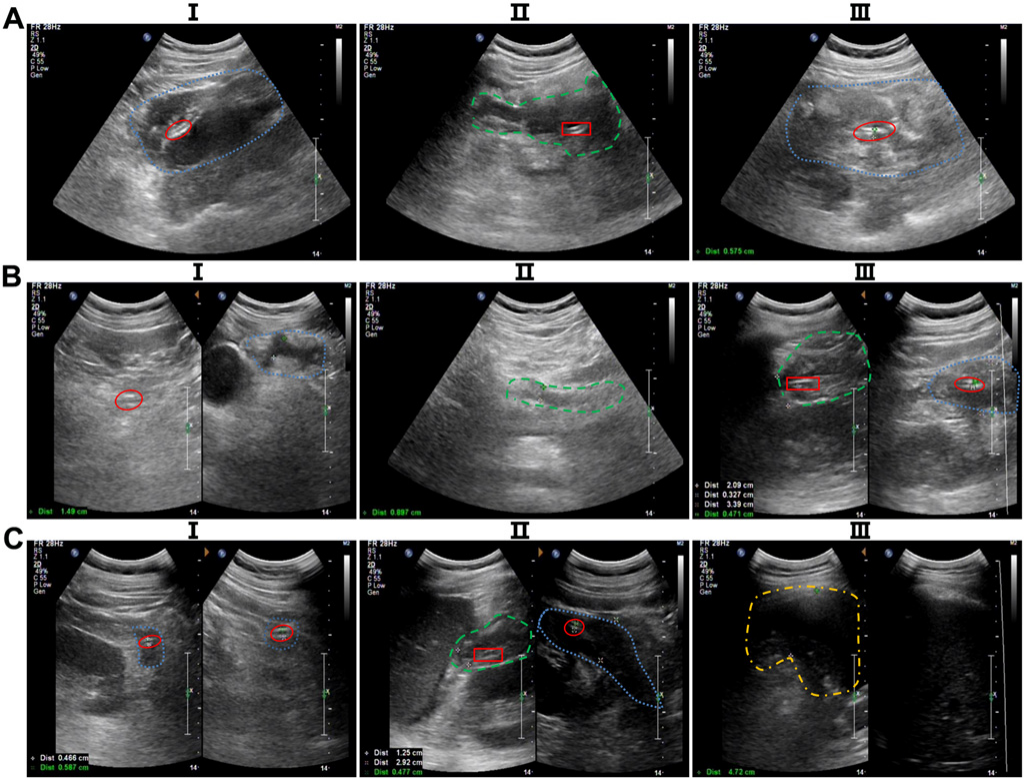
Ultrasonic images of fluid collections and drainage catheters at different time intervals after the interventions. (A) One day after drainage interventions: I, the amount of fluid collection of RPCS was large (blue dashed line) and was extracted by right APD intervention (red ellipse); II, the fluid collection of RPRR was widely distributed (green short line) and was drained by right PCD intervention (red rectangle); III, the amount of fluid collection of LPCS was also large (blue dashed line) and was drained by left APD intervention (red ellipse). (B) Ten days after drainage interventions, I, the amount of fluid collection of RPCS was greatly reduced (blue dashed line), and the fluid was egressed by right APD intervention (red ellipse); II, the fluid collection of the lesser omental bursa is shown by a short green line; III, the fluid collection of LPCS was also lessened in amount (blue dashed line) and was diminished by left APD intervention (red ellipse), and the fluid collection of LPRR decreased substantially (green short line) and was drained by left PCD intervention (red rectangle). (C) At 20 days after drainage interventions, I, the fluid collection of RPCS was almost gone (blue dashed line) and the right APD intervention (red ellipse) remained in place; II, the fluid collection of LPCS was slightly decreased (blue dashed line) and extracted by left APD intervention (red ellipse); meanwhile, the fluid collection of LPRR was greatly reduced (green short line), and the fluid was extracted by left PCD intervention (red rectangle); III, the amount of fluid collection in the left pectoral cavity was large (yellow short and dashed line), and the fluid was drained by extra-catheter intervention; in contrast, there was minimal fluid in the right pectoral cavity. RPCS = right paracolic sulci; LPCS = left paracolic sulci; RPRR = right pararenal region; LPRR = left pararenal region.
